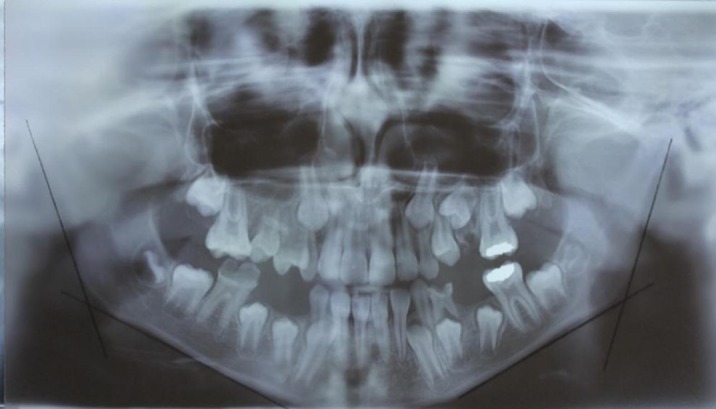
The gonial angle in panoramic radiography
